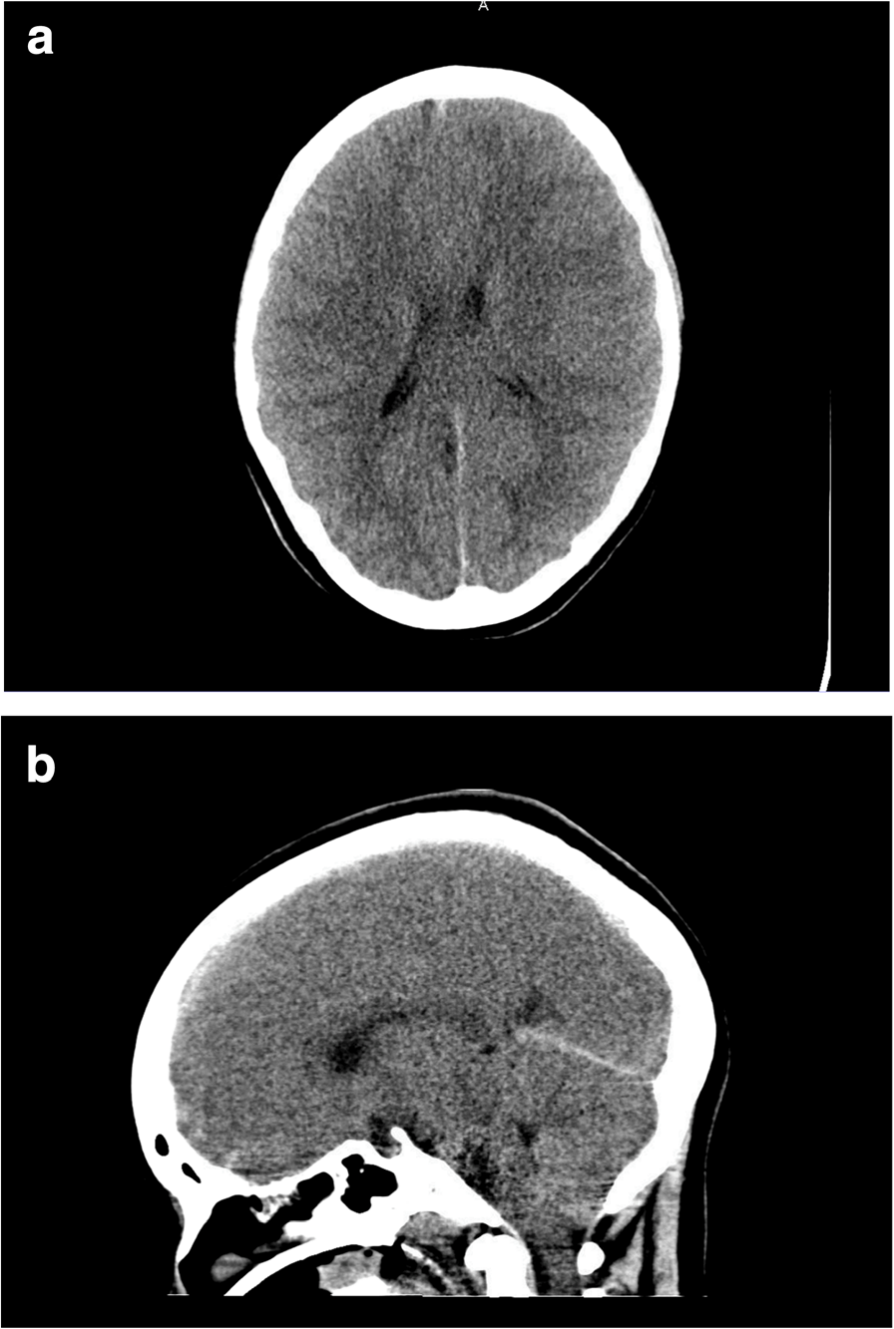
a Slice of CT brain showing cerebral edema. b Slice of CT brain showing suspected uncal herniation
